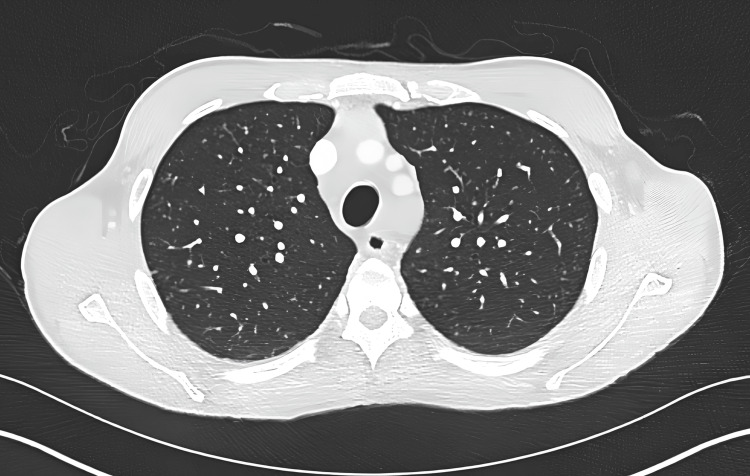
CT of the thorax (axial view) showing multiple tiny lung nodules bilaterally with the differential of calcification and miliary TB CT: computed tomography, TB: tuberculosis
